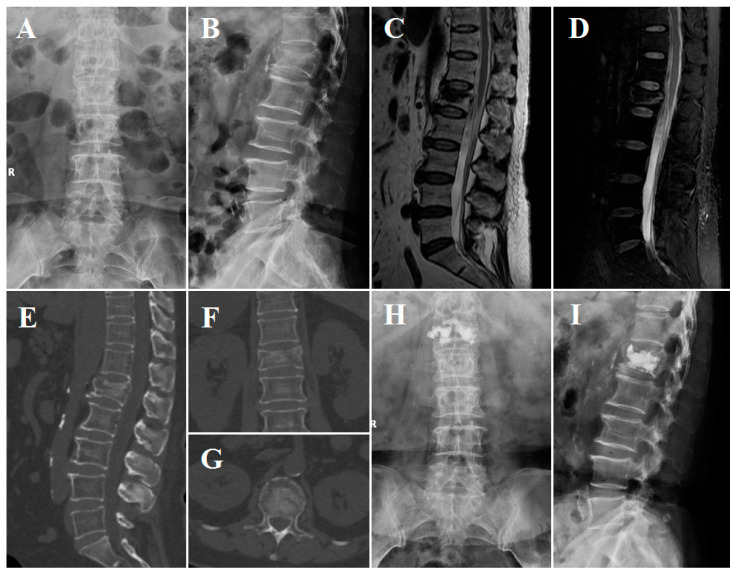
Imaging obtained from the patient described above. (A–D) Preoperative X-rays and MRI. (E–G) Preoperative CT showing fissures in the lateral and upper endplates of the fractured vertebral body. (H,I) Postoperative lumbar X-rays obtained one day after PKP.
